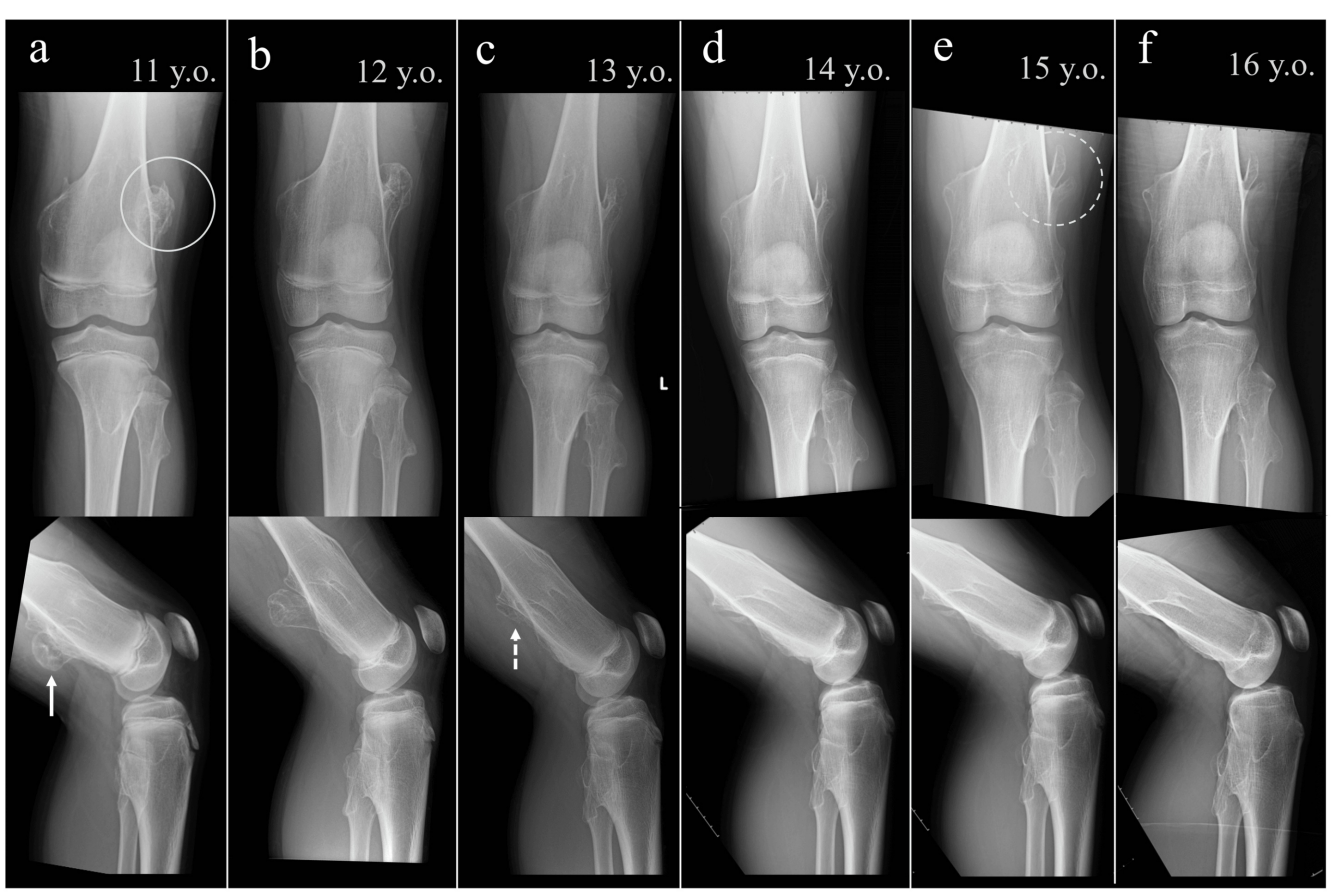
Serial radiographs of the left knee from ages 11 to 16 (a) Age 11: Sessile osteochondromas are visible on both the lateral (white circle) and posterior (white arrow) aspects of the distal femur. (b) Age 12: Both lesions increased in size. (c) Age 13: The posterior lesion regressed (dotted arrow). (d) Age 14: The lateral lesion showed a slight decrease in size (mild regression). (e) Age 15: The lateral osteochondroma transitioned from a sessile to a pedunculated morphology (dotted circle). (f) Age 16: Complete physeal closure was confirmed.
